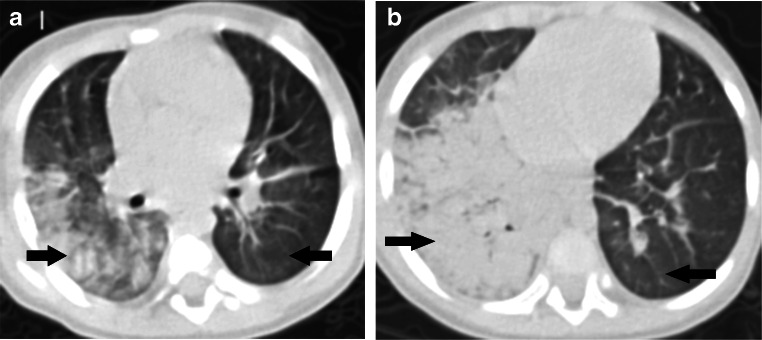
A 1-year old critically ill boy with COVID-19 with diarrhea, fever and a history of exposure. a, b Axial thin-section non-contrast CT images show diffuse ground-glass opacities bilaterally, extensive consolidation at the right lung and reticulation bilaterally (*arrows*)
